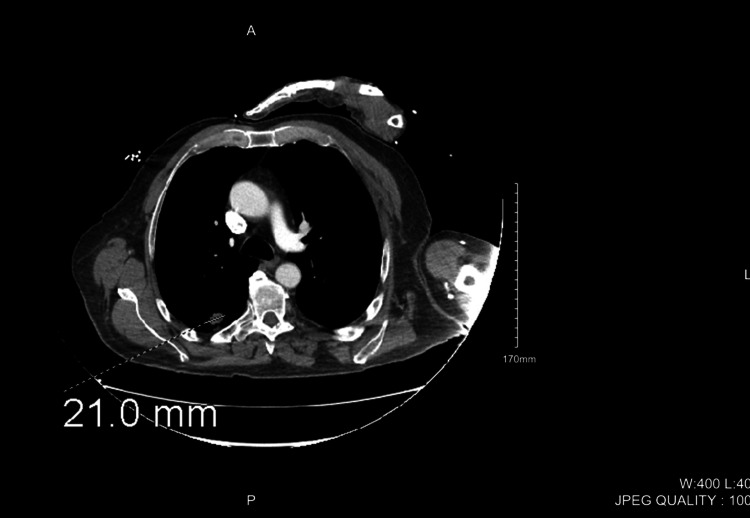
CT of the lung showing one of two apical right lower lobe tumors CT: computed tomography
